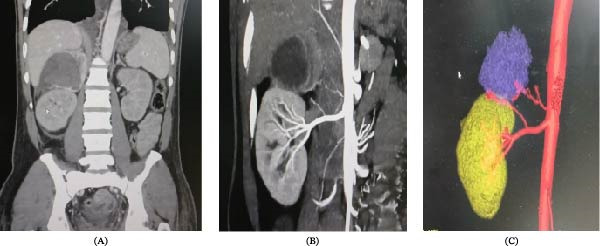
(A) Abdominal CT (adrenal region), coronal abdominal CT: A heterogeneous right suprarenal mass is visible, abutting the kidney and liver. (B) Contrast‐enhanced images show heterogeneous enhancement with areas of necrosis/calcification and possible capsular/lateral wall breach. Features strongly suggest adrenal neoplasm (pheochromocytoma). Right (3D reconstruction). (C) Demonstrates vascular relations of the adrenal mass. The yellow/purple segmentation highlights tumor margins and their relation to renal vessels and inferior vena cava, essential for preoperative surgical planning.
